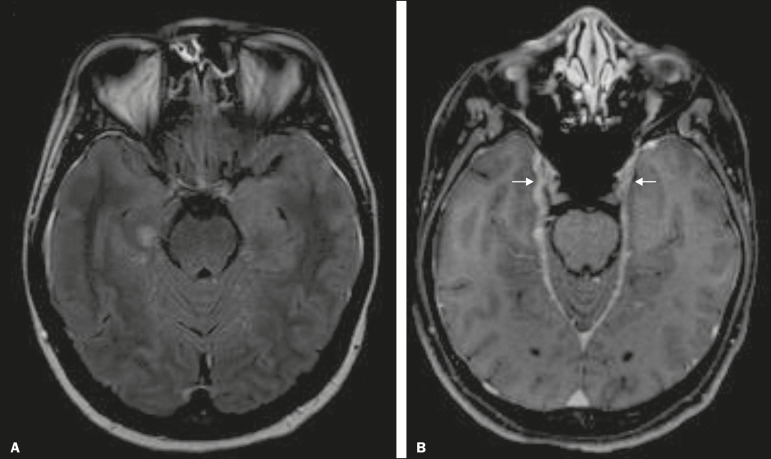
A 35-year-old, HIV-positive female patient who presented with a one-week history of fever and left frontoparietal headache. Cerebrospinal fluid analysis showed high protein levels, lymphocytic leukocytosis, reduced glucose, and increased adenosine deaminase, favoring a diagnosis of neurotuberculosis. Axial FLAIR-weighted and contrast-enhanced axial T1-weighted MRI sequences (A and B, respectively) showing thickening of the dura mater (arrows), which was more pronounced in the right temporal region, where the FLAIR-weighted shows a change in the signal. The patient showed symptom improvement, the follow-up examinations performed one year after the onset of symptoms showing resolution of the radiological aspects (images not shown).
